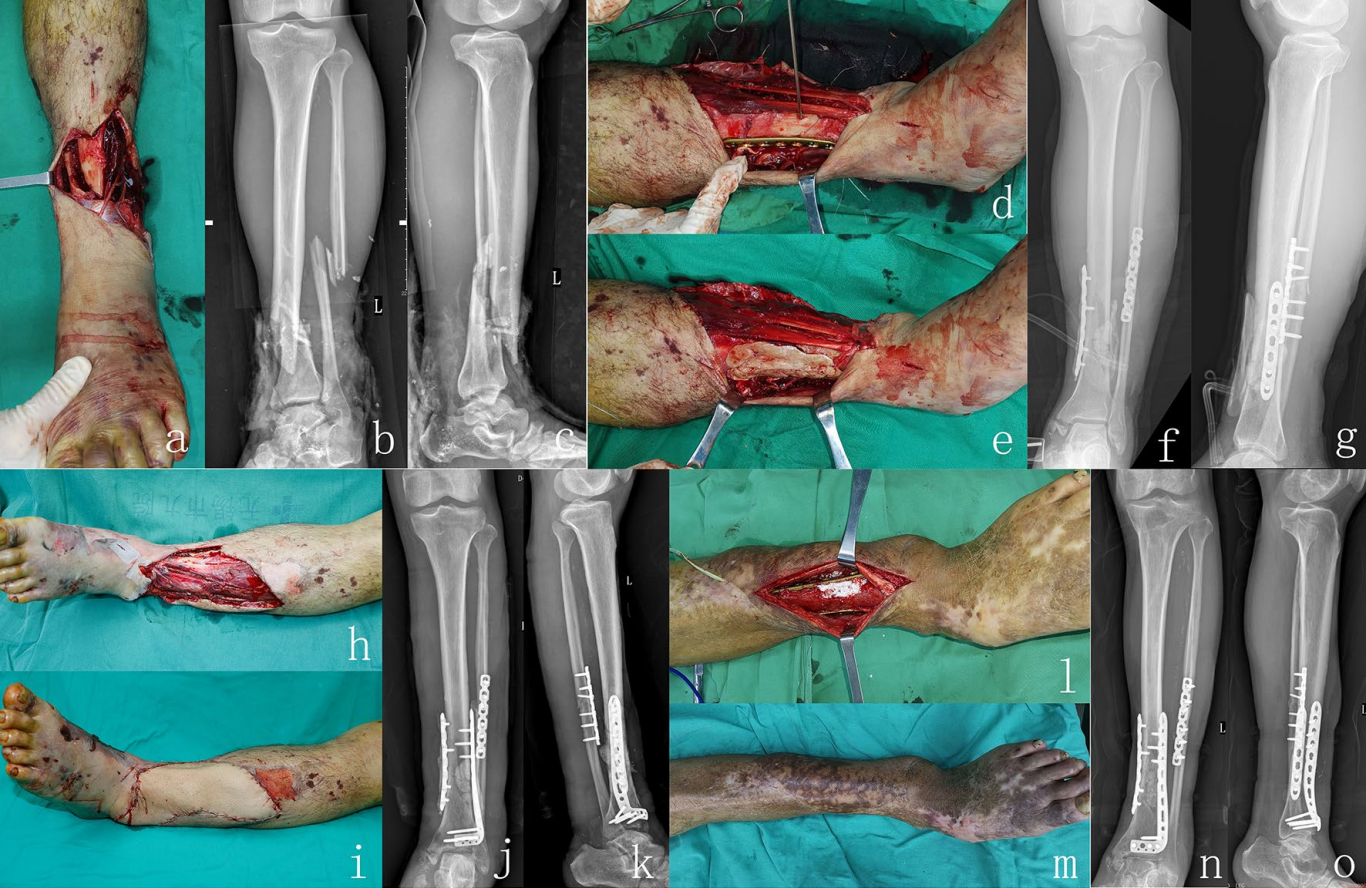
An example of an injury in a 64-year-old male patient. (a) Visual inspection of the injury showing anterior and lateral wounds on the lower part of the left. (b) Anteroposterior (AP) radiographs showing comminuted fractures in the middle and lower part of the tibia and the middle part of the fibula. (c) lateral radiographs of the fracture. (d) Fixation of the fracture with the locking plate. (e) The surface of the locking plate was completely covered with antibiotic PMMA cement. (f) and (g) The patient’s AP and lateral radiographs showing the fracture is fixed by antibiotic cement-coated locking plate. (h) The irregular defect was located on the patient’s left side. (i) A free flap covering the reconstructed soft-tissue. (j) and (k) The patient’s AP and lateral radiographs showing fixation of the fracture with an antibiotic cement-coated locking plate and anatomically-locked compression plate. (l) the bone cement was removed, and the defect was filled with autogenic cancellous bone. (m) appearance at 14-month follow-up. (n) and (o): The AP and lateral radiographs showed fracture healing at 14 months after surgery. AP, anterior posterior.
